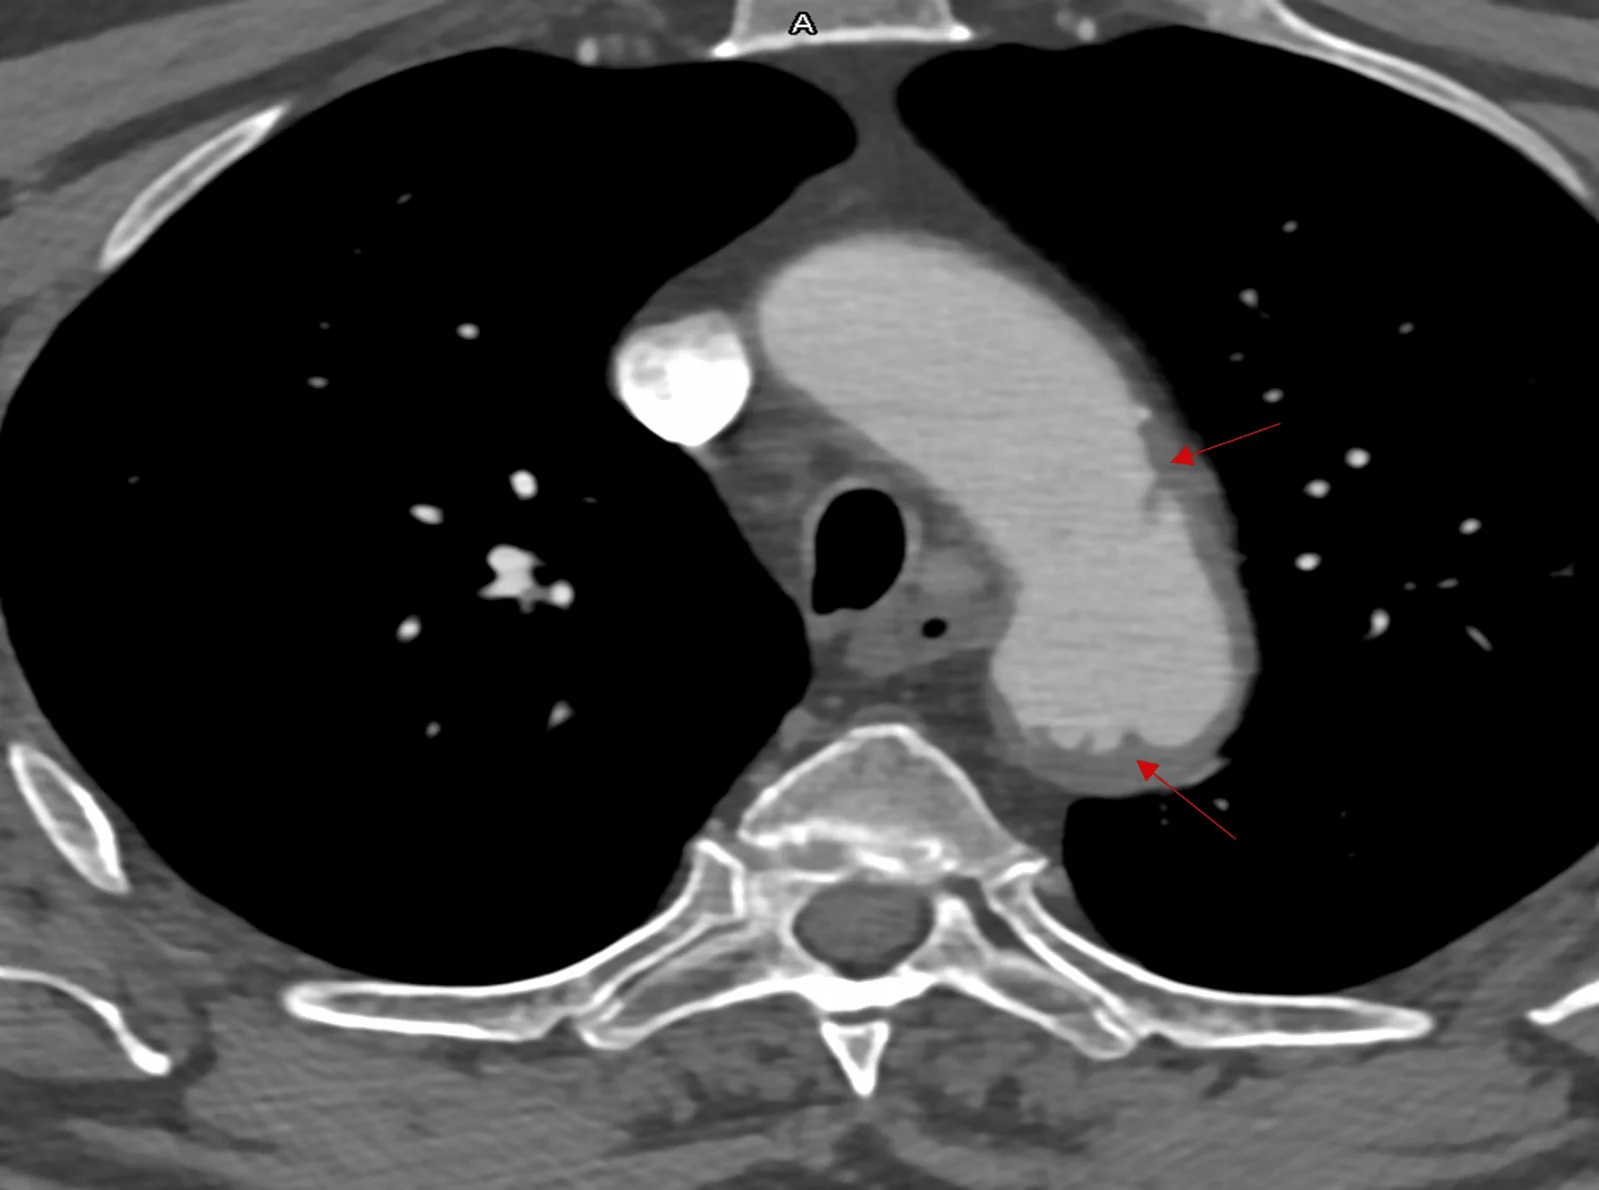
Computed tomography angiography showing an irregular atheromatous plaque involving the aortic arch and aortic isthmus (red arrows)
